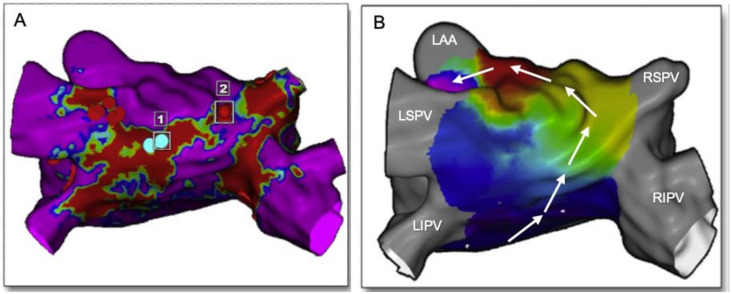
Usefulness of cardiac MRI in redo procedures of atrial fibrillation and atrial flutter ablation. (A): MRI-LGE of the left atrium in a patient with previous ablation of the roof presenting with atypical flutter. Red areas represent scar due to previous ablation (blue dots = 1). In point 2 (red dot), there is a portion of healthy tissue (purple) between the roof line and the RSPV. (B): Activation mapping during flutter with CARTO system depicts a circuit directed upwards along the posterior wall and crossing the gap identified at the MRI (white arrows). One-shot ablation in point 2 interrupted the roof-dependent atrial flutter. LAA: left atrial appendage, LSPV: left superior pulmonary vein, LIPV: left inferior pulmonary vein, RSPV: right superior pulmonary vein, RIPV: right inferior pulmonary vein. Modified from [49].
